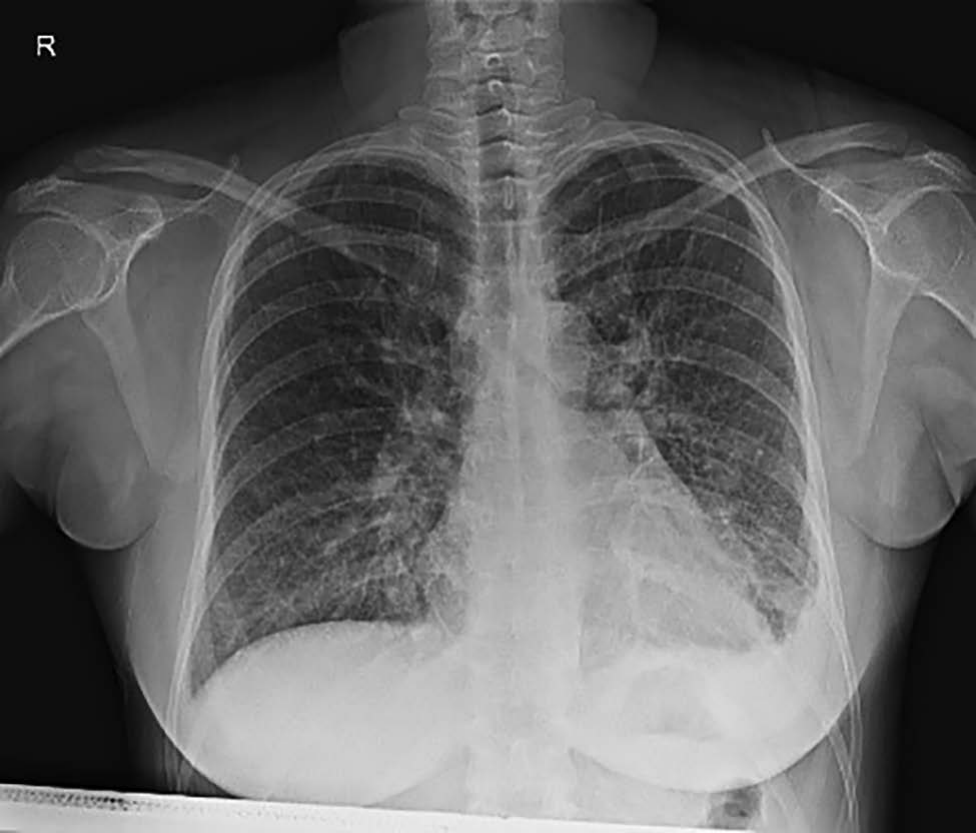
Chest X-ray at GA 36 weeks increased left pleural-effusion.
